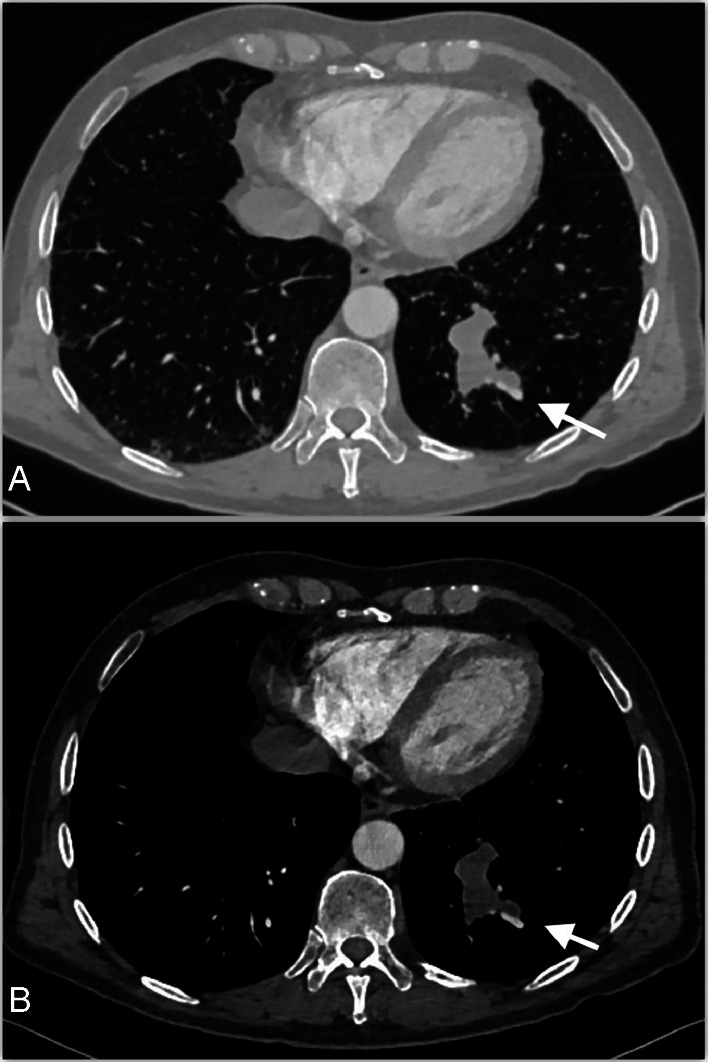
(A,B): CT with contrast axial lung window (A) and mediastinum window (B). (A) Multinodular lesions were appreciable in the left lower lobe with hyperinflation (air trapping) of adjacent lobules (arrow). (B) CT showed multinodular confluent markedly hypodense lesions with no air fluid levels in the left lower lobe (arrow).
